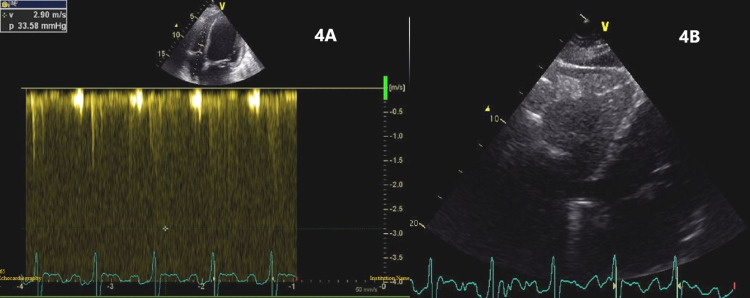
Continuous wave Doppler across the tricuspid valve showing mildly elevated right ventricular pressure (A) and the inferior vena cava was not dilated (B).
